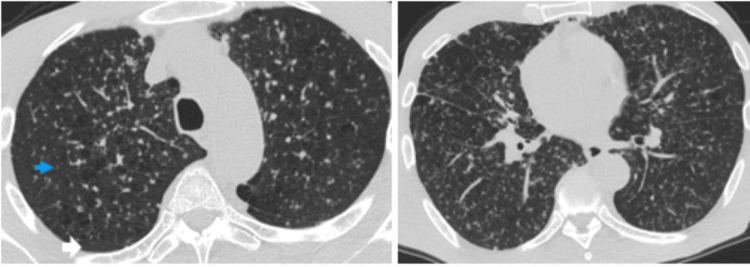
Chest CT of patient one The image shows miliary pulmonary nodules (blue arrowhead). There was no evidence of pulmonary masses, but the background lung shows emphysema with few bilateral upper lobe centrilobular bullae in and small subpleural bullae in the left apex (white arrow). No enlarged mediastinal or hilar nodes or fibrocavitatory changes were present.
